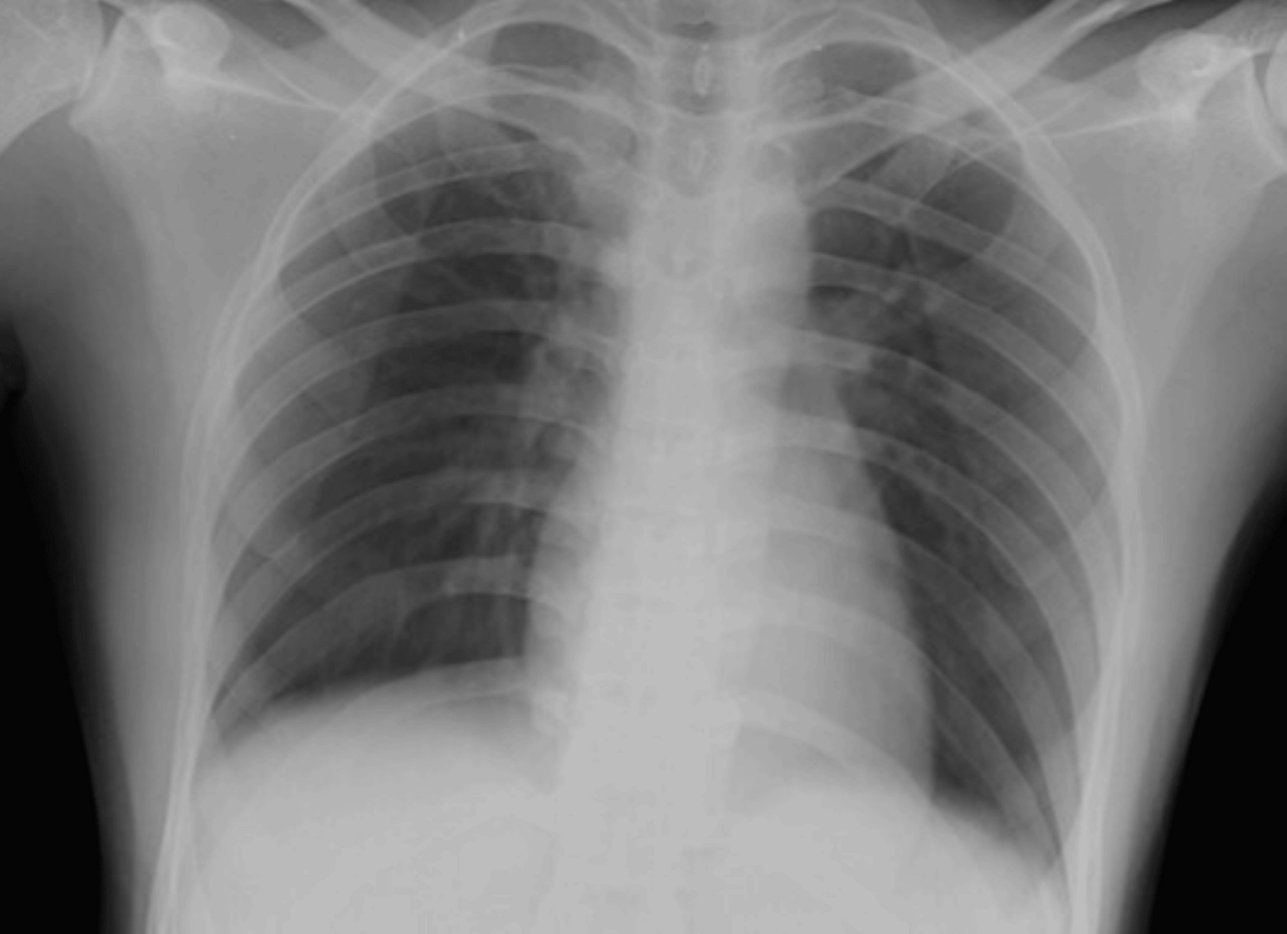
The supine chest radiograph (anteroposterior (AP) view) of a 23-year-old female patient showed no obvious radiopacities in both lung fields. Radiographic disease score was 0.
